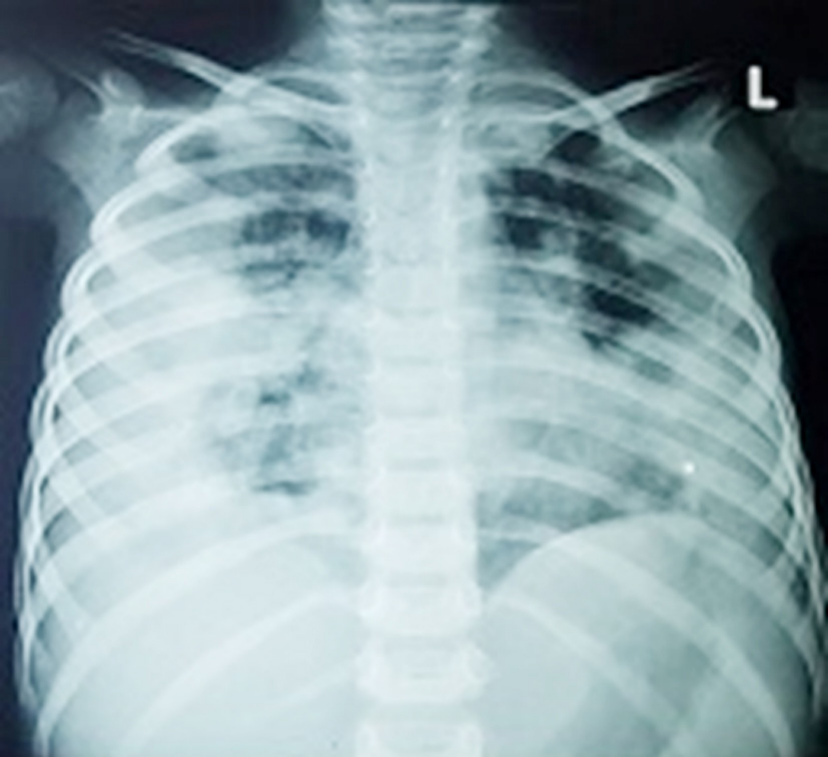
Chest X-ray image of a COVID -19 positive 56 years old male with no travel/contact history, presented with high grade fever, sore throat and cough for two days, showing bilateral mid and lower zones homogenous consolidation in peripheral distribution (L>R) along with Obscuration of both CP angles- Findings fall in the category of indeterminate for COVID-19.
